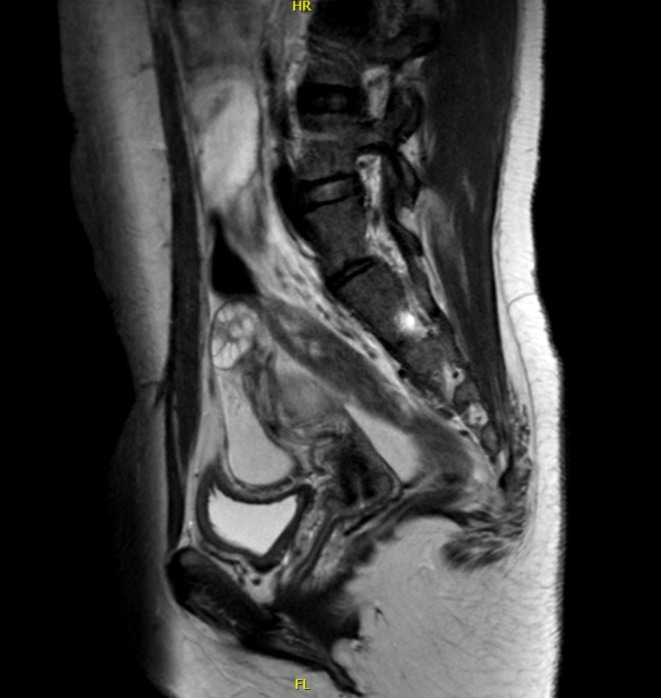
Multiparametric pelvic MRI, sagittal T2W TSE HR sequence. The most cranial part of the ureter in left renal lodge communicates with an oval component with irregular morphology and multiloculated thickened walls, compatible with pluriconchambered abscess formation.
